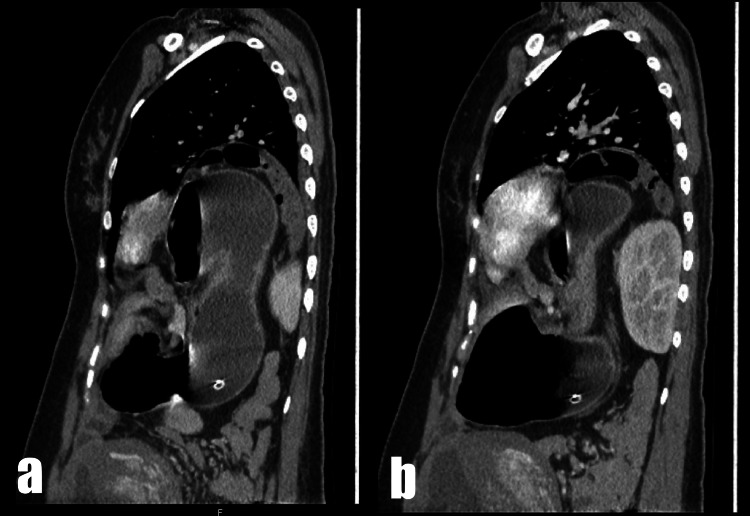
CT thorax and upper abdomen sagittal sections showing (a) posterolateral diaphragmatic herniation of stomach and (b) ectopic left kidney. CT, computed tomography
